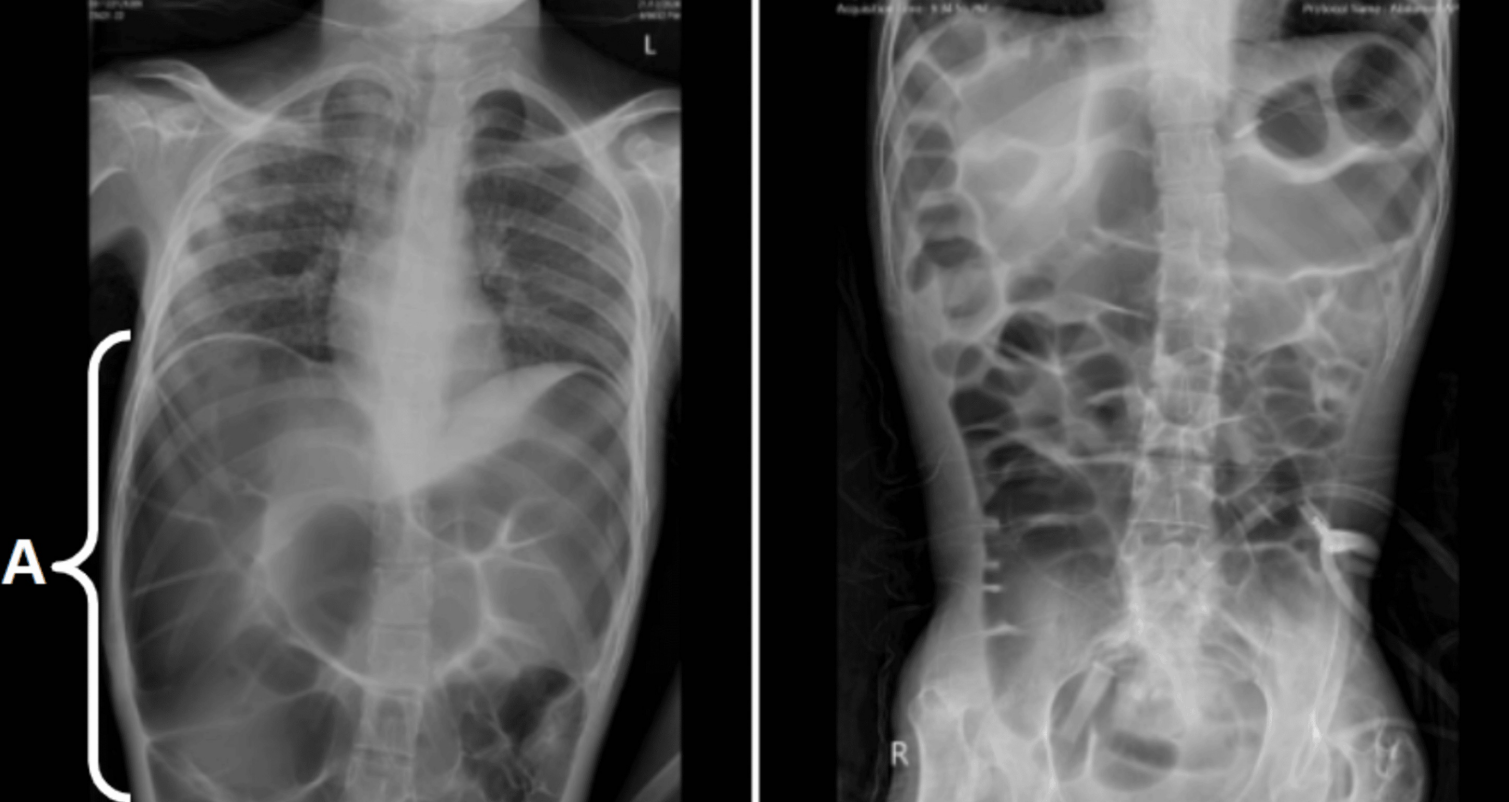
Erect chest radiograph (left) and abdominal radiograph (right) The erect chest radiograph shows dilated large bowel loops under both hemidiaphragms (marked by A), suggestive of proximal colonic obstruction. The abdominal radiograph demonstrates gross colonic dilatation without evidence of pneumoperitoneum.
